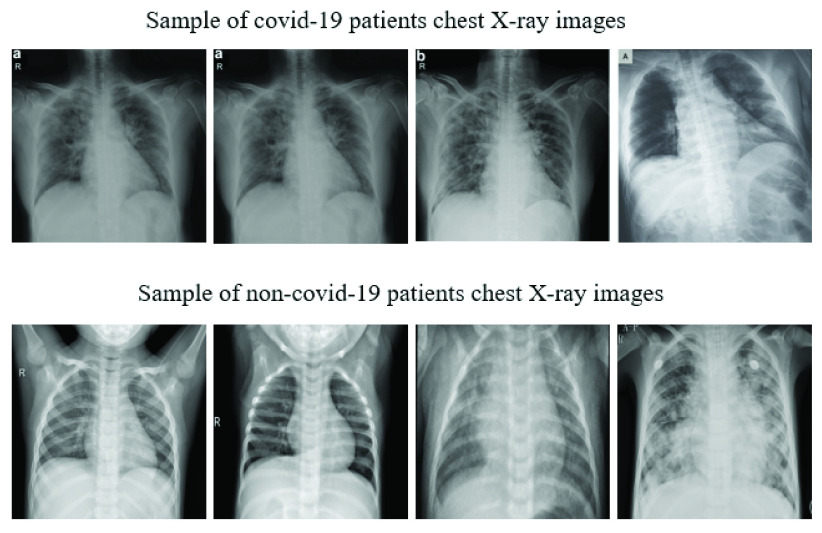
Representative samples of chest X-ray images from the open source data repositories [43] used in our proposed studies.
